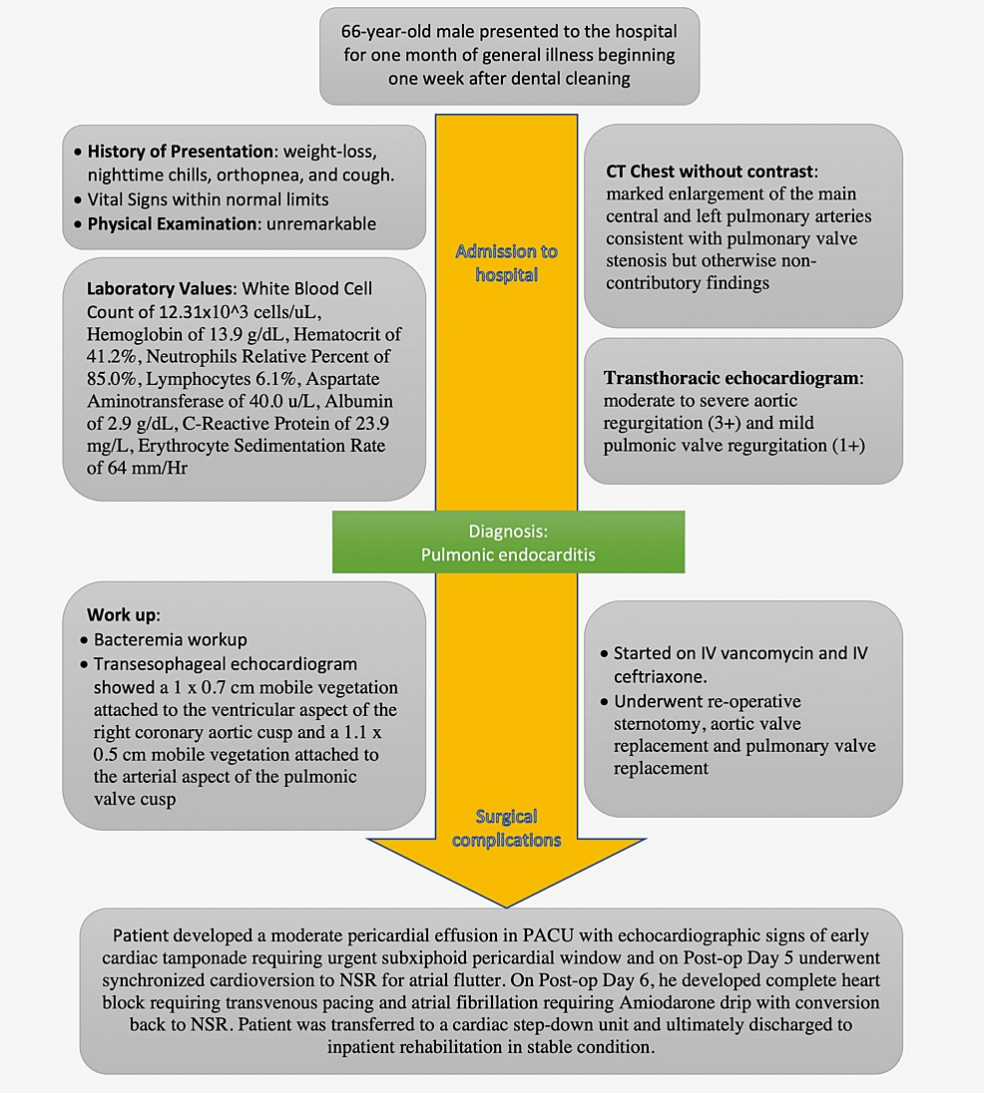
Timeline highlighting the case events. Figure credit: Kalei Lopez CT, computed tomography; IV, intravenous; NSR, normal sinus rhythm; PACU, postanesthesia care unit
